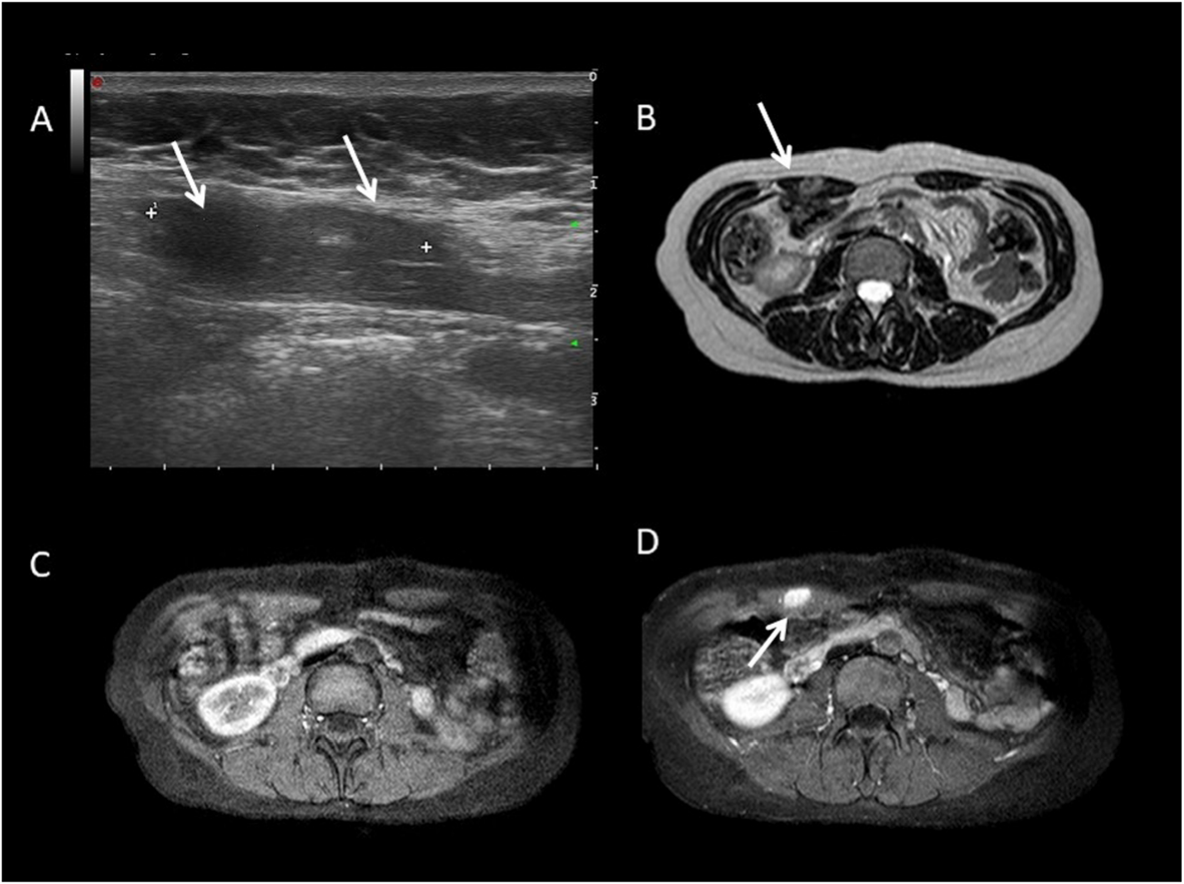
Young female patient with a positive history for pregnancy and cesarean section. Abdominal wall DT (white arrow) appears as homogeneously hypoechoic masses at US examination (a). On MRI, it is characterized by T2 hyperintensity on T2wi (b) and homogeneous enhancement on fat sat T1wi (d). Fat sat T1wi before contrast (c)
